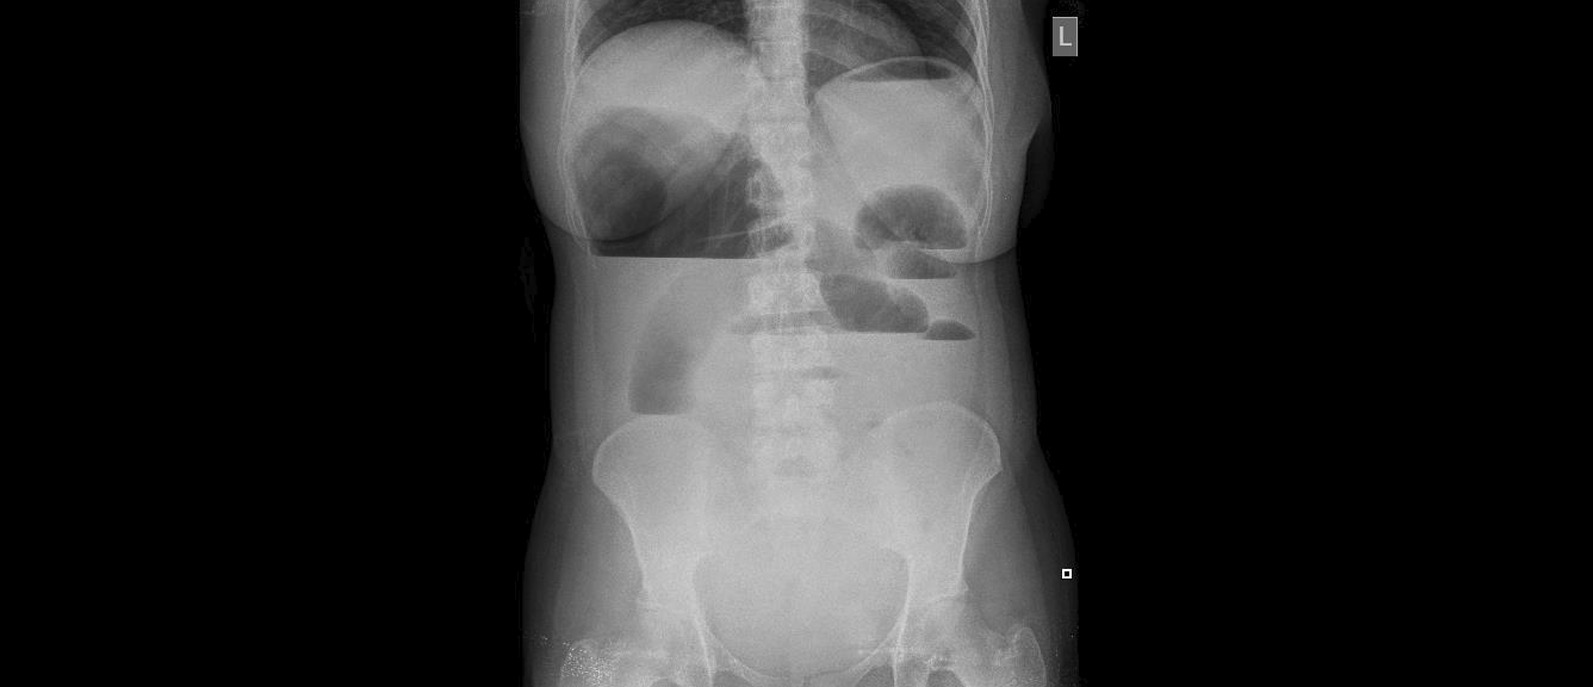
Erect abdominal radiograph showing dilated small bowel air-fluid levels with distended colon in right-upper quadrant
